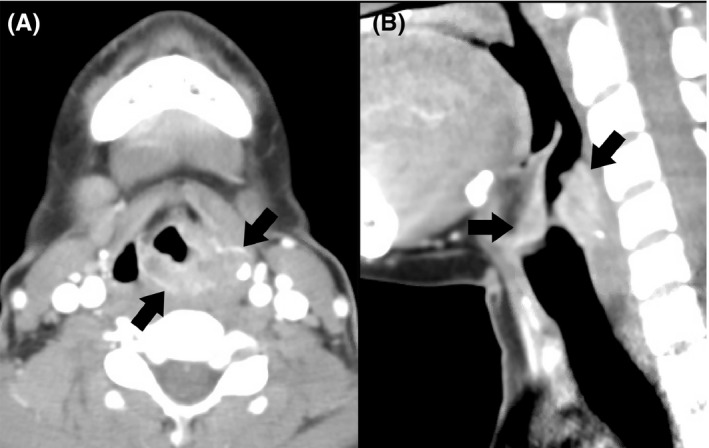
Axial (A) and sagittal (B) contrast‐enhanced axial CT images demonstrate an infiltrative, heterogeneously enhancing mass centered in the supraglottis involving both aryepiglottic folds with obliteration of the left piriform sinus (arrows). There is partial infiltration of the paraglottic fat
